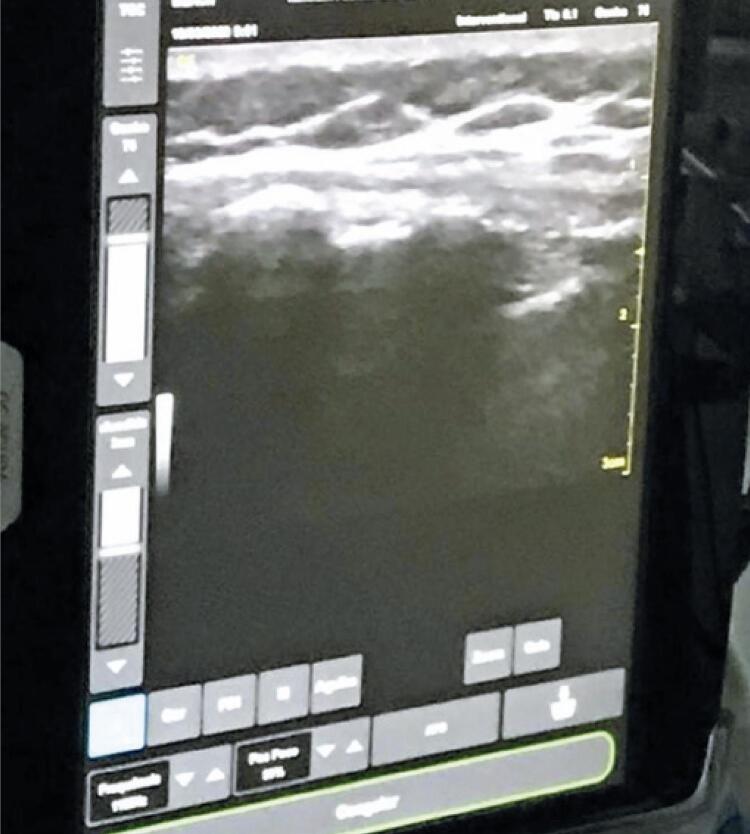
Ultrasound with linear transducer for locoregional anesthesia
